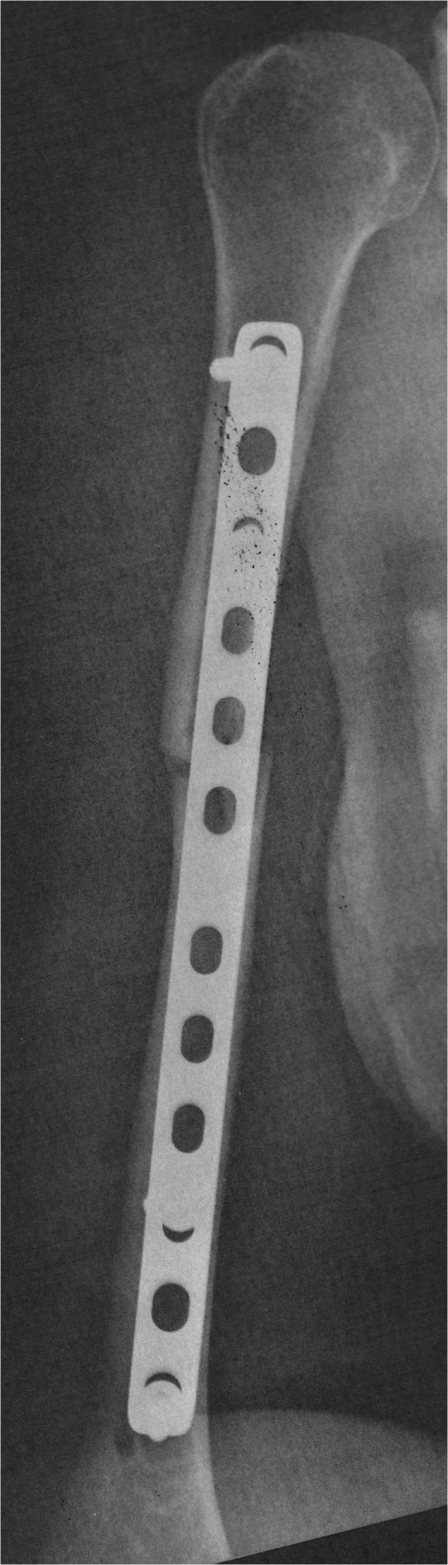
Postoperative radiography.
